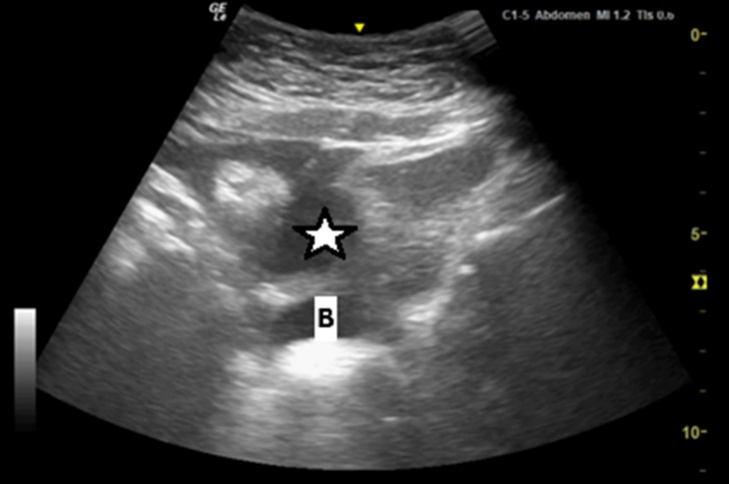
Point of care ultrasound (POCUS) image of pelvis in transverse plane using the curvilinear 5-12 MHz probe, demonstrating a moderate amount of free fluid (*) anterior to the bladder (B).
